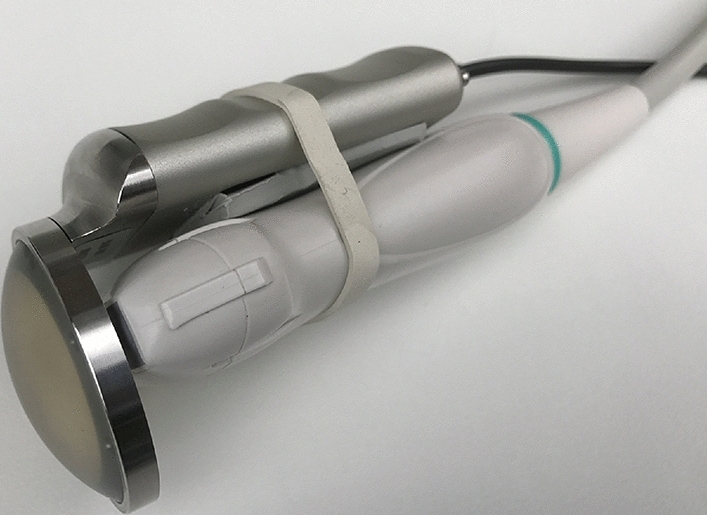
Veinpress system mounted on an ultrasound probe (adapted with permission from Bloch et al. [24])
